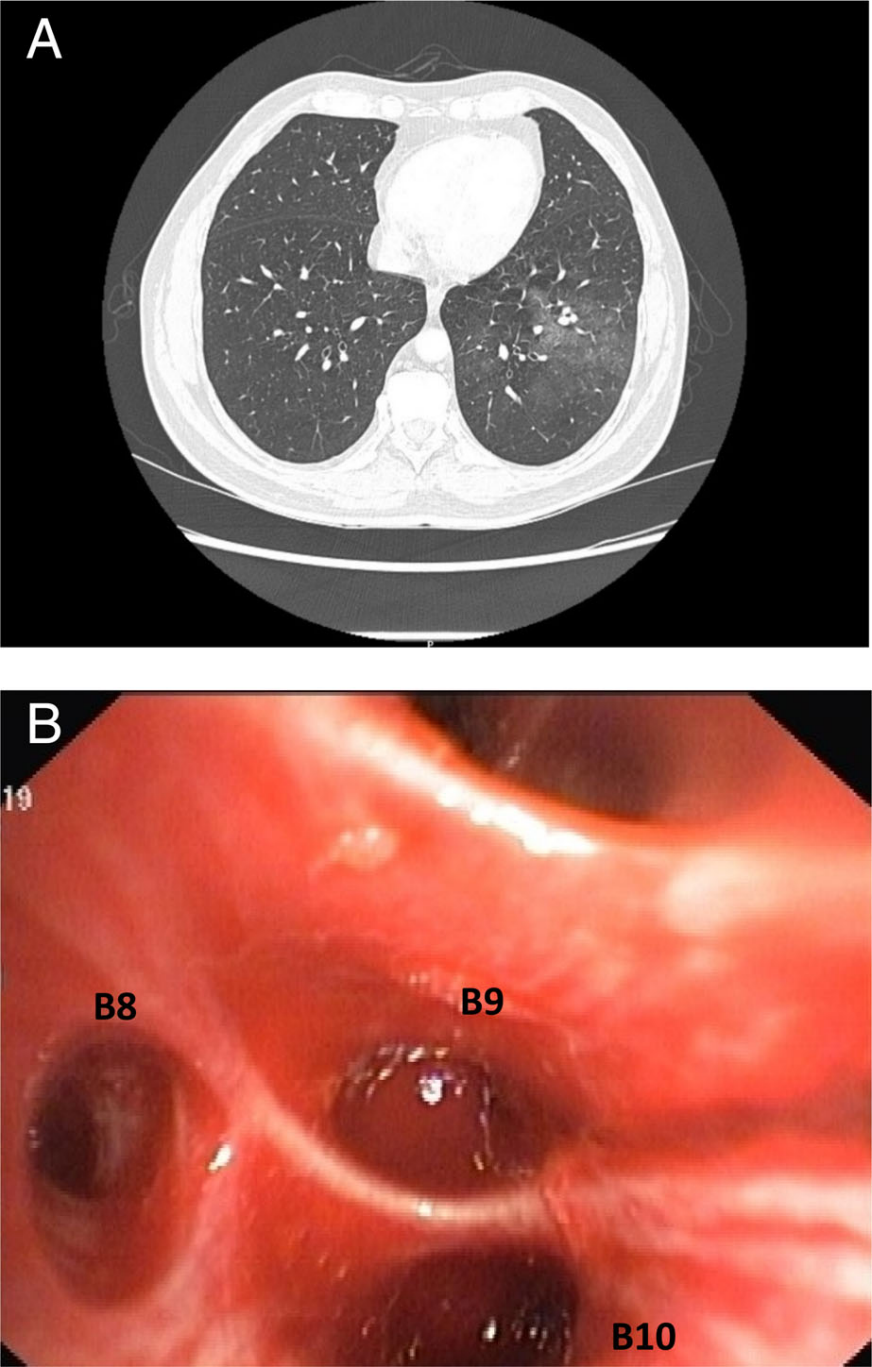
(A) Computed tomography (CT) scan showing ground‐glass opacity in the left lateral basal segment indicating the site of bleeding. (B) Bronchoscopy image showing pools of blood in the B9/B10 segments of the left lower lobe.
